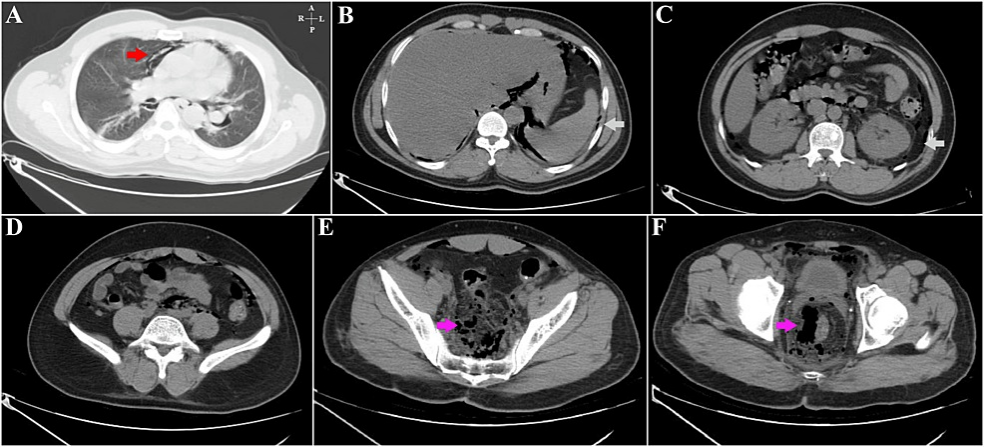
Patient 2 - CT images of the abdomen in the axial plane Figure 4A – Lung window; streaky air densities (red arrow) are seen anterior to the pericardial space outlining the pulmonary vasculature bilaterally. Figure 4B – Air is seen outlining the descending abdominal aorta and the medial aspect of the spleen with air lucencies (cyan arrow) also seen in the gastro-hepatic space, the anterior pre-peritoneal space, and adjacent to the right diaphragmatic crus. Figure 4C – Axial image at the level of the L2 vertebral body showing air foci (cyan arrow) in the extra-peritoneal space. Figure 4D – At the level of the L5 vertebral body with air foci seen in the extra-peritoneal space. A few air foci are seen anterior to the left psoas, which is the major muscle. Figure 4E – Axial place image through the sacro-iliac joints showing abundant air foci (pink arrow) in the para-rectal space extending superiorly. Figure 4F – At the level of the femoral heads, the air is noted (pink arrow) in the para-rectal space, with few air foci seen in the anterior pre-peritoneal space.
